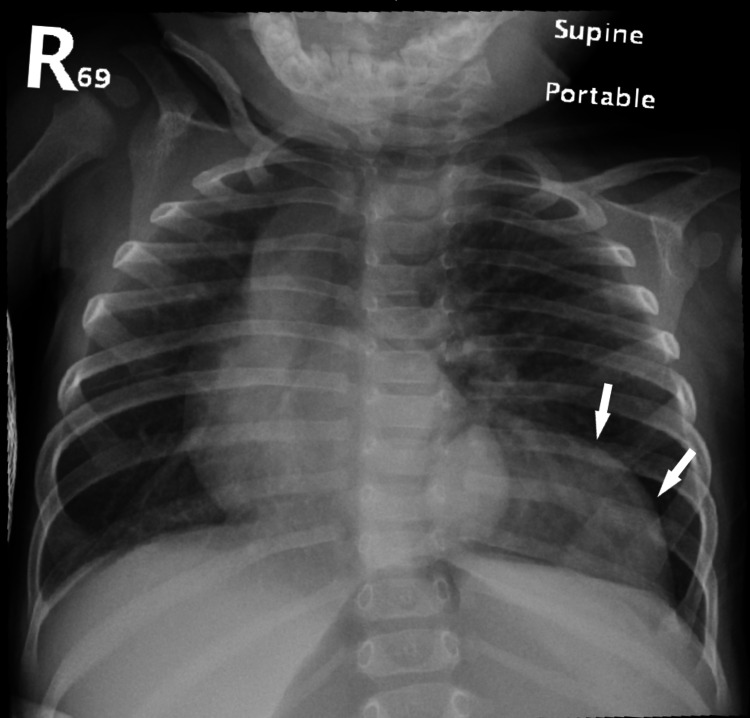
Chest radiography Chest X-ray showing left lower lung opacity (arrows).
